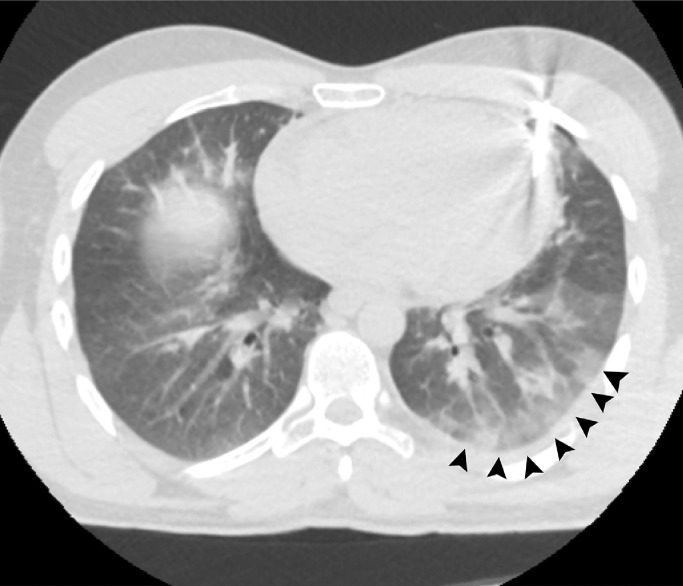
Lung window of the chest CT demonstrated subsequent pulmonary hemorrhage (black arrowheads).
